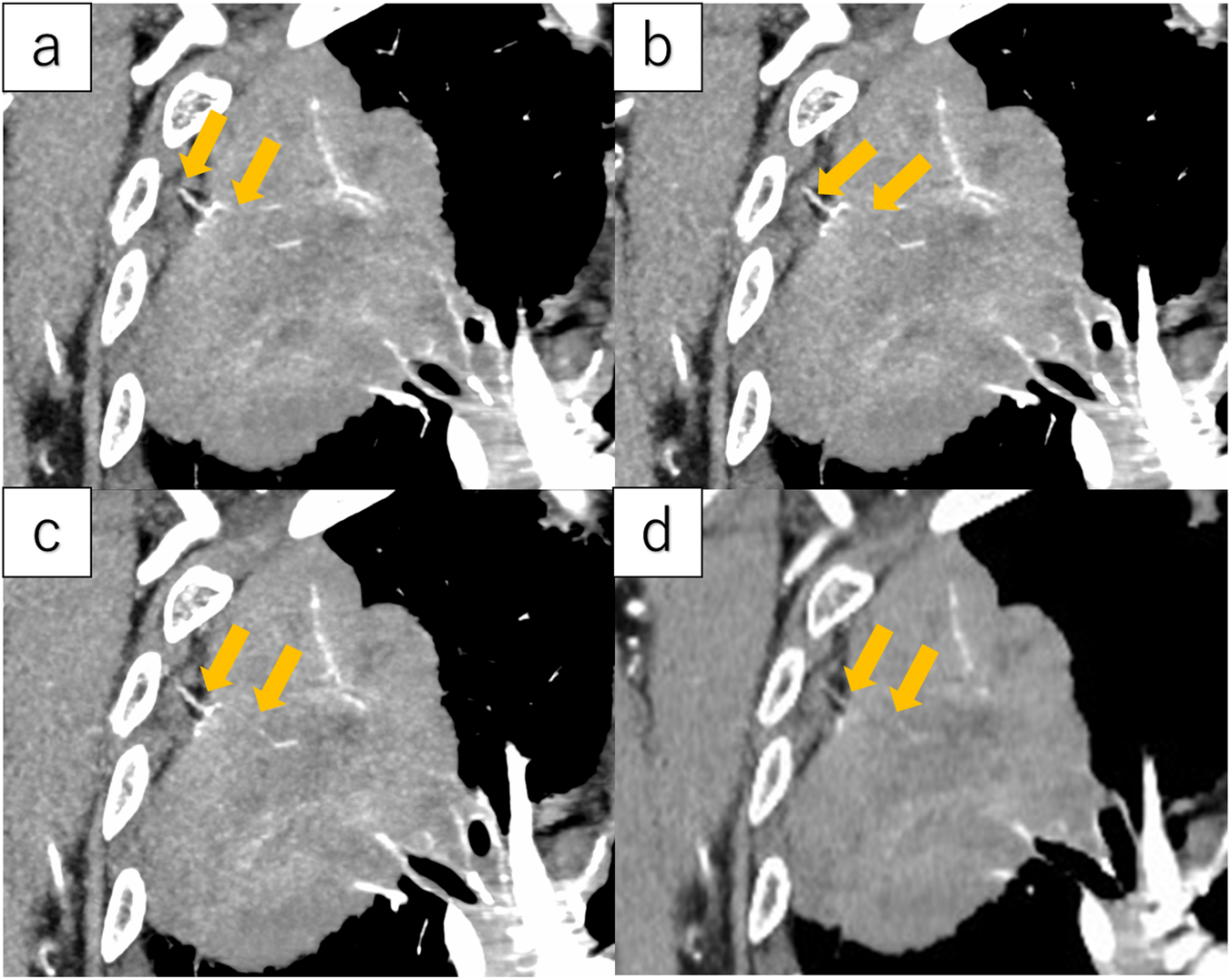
Maximum intensity projection created from ultra-high-resolution CT images Ultra-high-resolution CT images of a 79-year-old female showing adenocarcinoma infiltrating the chest wall in the right upper lobe. a, b, and c are coronal sections arranged ventral to dorsal. d is a conventional HRCT image. The tumor diameter was 85 mm and showed chest wall vessel involvement in subpleural lung cancer (arrow). The postoperative pathology assessment confirmed parietal pleural invasion of the tumor
